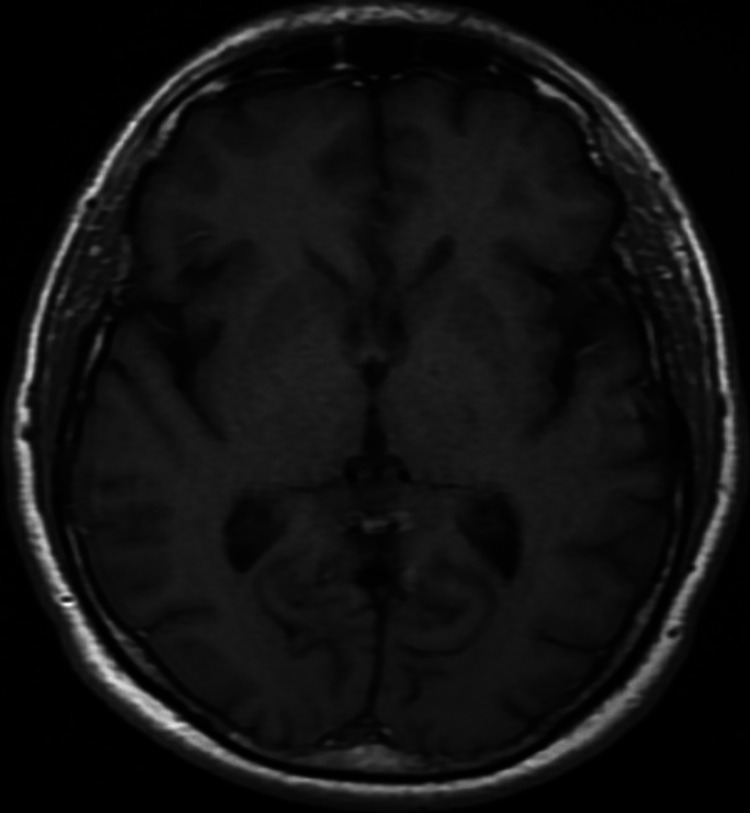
Normal magnetic resonance image of the brain
